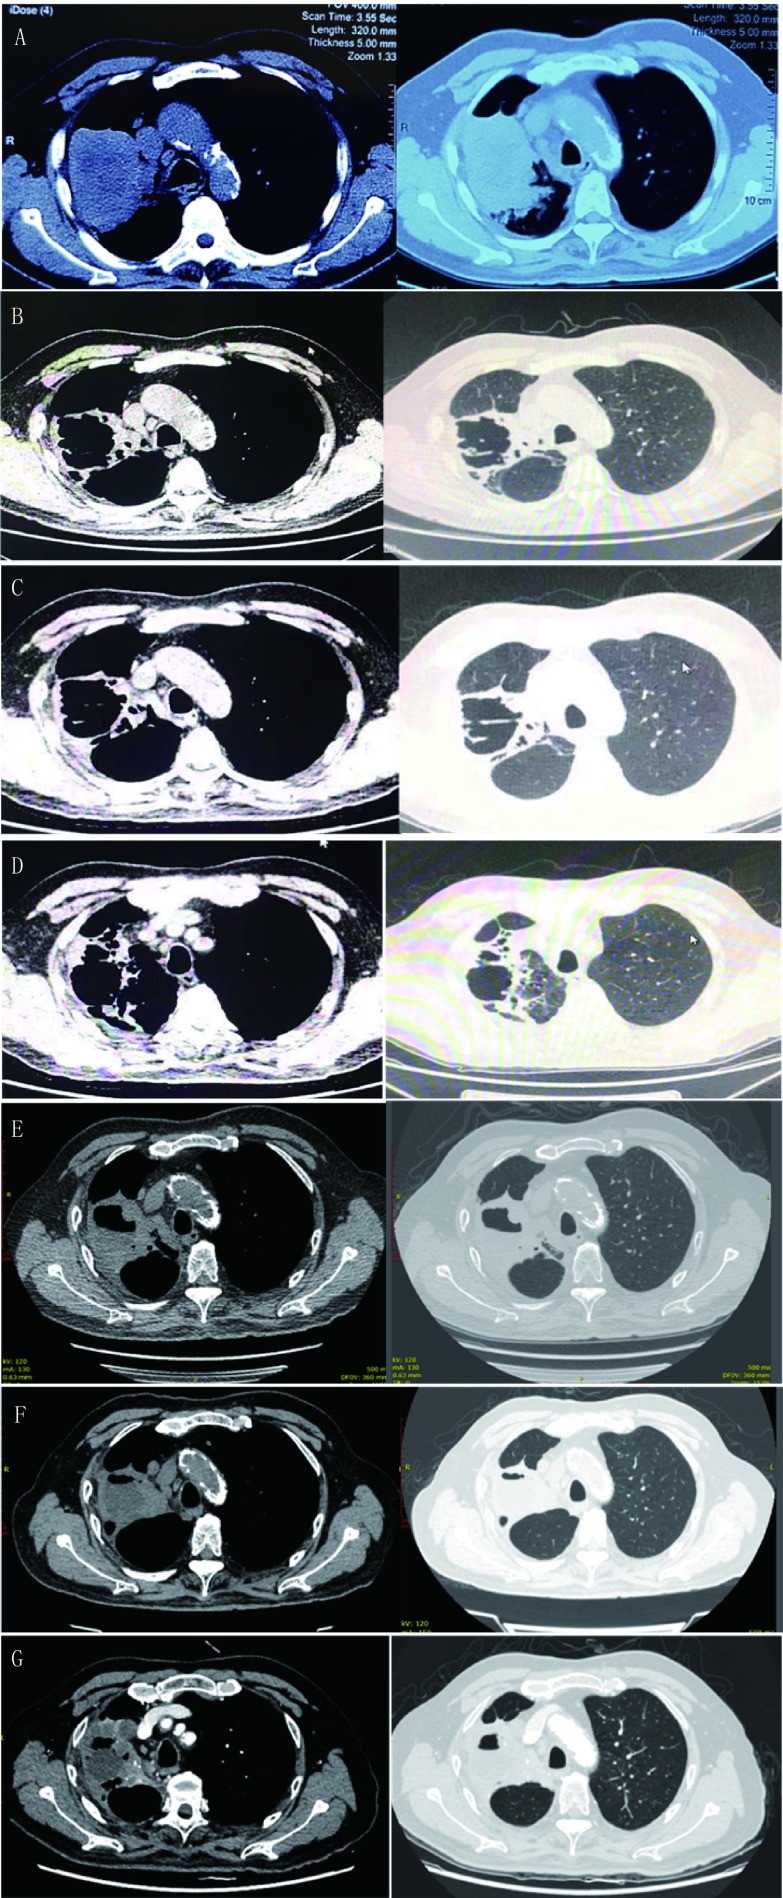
历次胸部CT扫描图像。A：2018年7月25日，右上肺肿瘤（长径84.8 mm）伴阻塞性肺炎，纵隔肺门淋巴结增大；B：2018年9月26日，右上肺肿瘤（长径54 mm）伴空洞形成；C：2018年10月18日，右上肺肿瘤（长径48 mm）；D：2018年12月18日，癌性病灶及空洞维持稳定；E：2019年4月11日，癌性病灶维持稳定空洞缩小；F：2019年7月1日，癌性病灶维持稳定空洞进一步缩小；G：2019年10月12日，癌性病灶及空洞维持稳定。 CT images of the chest. A: right upper lung tumor (major axis 84.8 mm) with obstructive pneumonia and mediastinal lymph node enlargement (Jul 25, 2018); B: right upper lung tumor (major axis 54 mm) with cavitation (Sep 26, 2018); C: right upper lung tumor (major axis 48 mm)(Oct 18, 2018); D: Cancer and cavity remained stable (Dec 18, 2018); E: cancer kept stable and cavity became smaller (Apr 11, 2019); F: Cancer kept stable and cavity shrinks further (Jul 1, 2019); G: cancer and cavity remained stable (Oct 12, 2019). CT: computed tomography.
